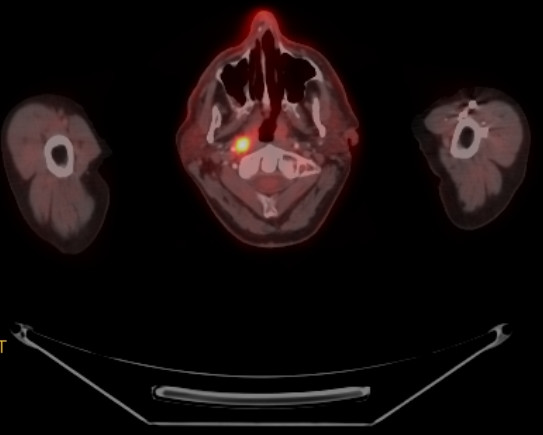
FDG-PET/CT scan of a patient with nasopharyngeal cancer. Transverse slice demonstrating FDG-positive primary site
